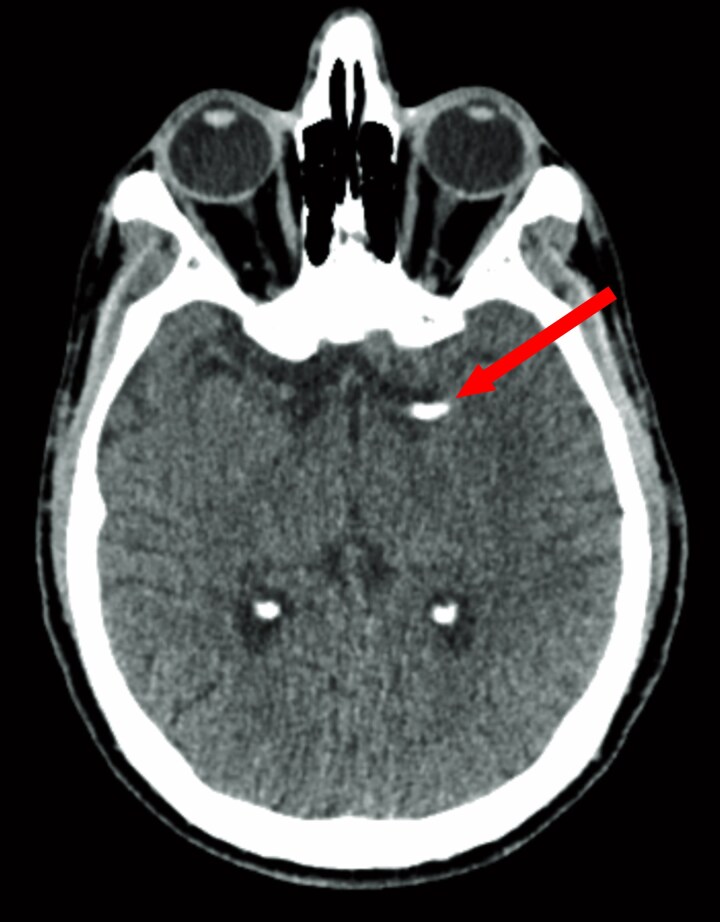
Brain CT findings. Non-contrast CT demonstrated a hyperdense intraluminal focus in the proximal M1 segment of the left MCA (arrows), measuring ∼850 HU compared with 42 HU in the contralateral M1 and ∼1350 HU in the adjacent skull table. Multi-planar reconstructions confirmed the focus centred within the lumen. CT angiography co-localized this hyperdensity with an intraluminal filling defect, consistent with a calcified embolus.
